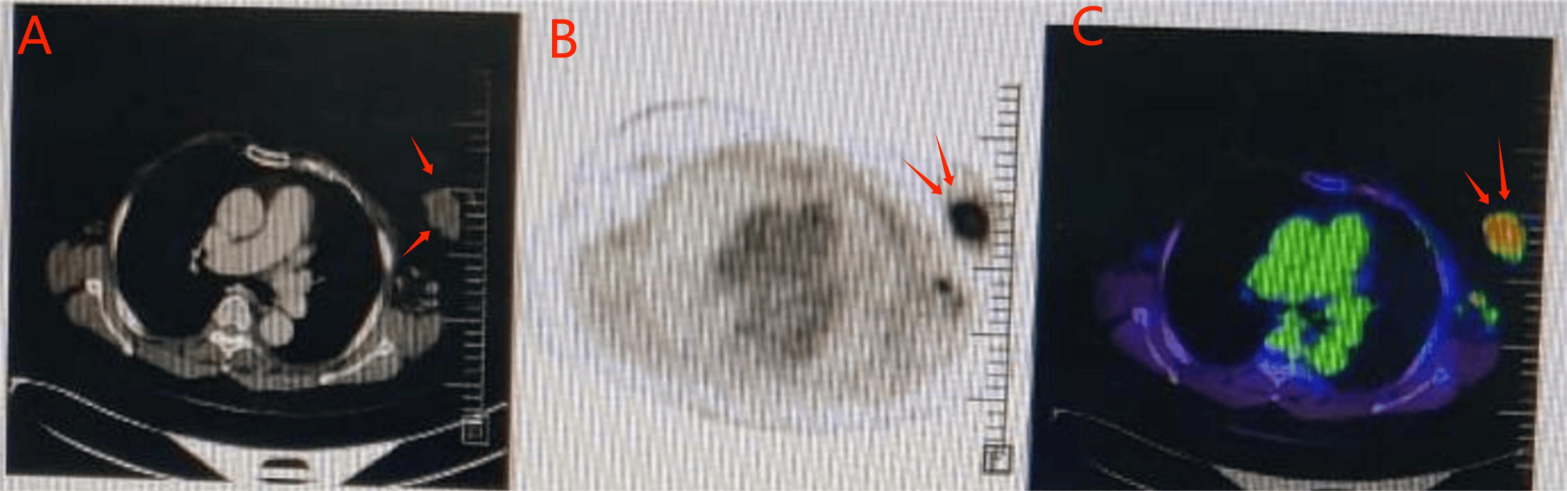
PET-CT demonstrating an FDG-avid irregular soft tissue density lesion in the left breast (arrows) with no evidence of distant metastasis A: axial CT image shows the left breast lesion; B: PET image highlights intense FDG uptake; C: fused PET-CT image confirms the metabolically active breast mass without distant lesions. FDG: fluorodeoxyglucose
